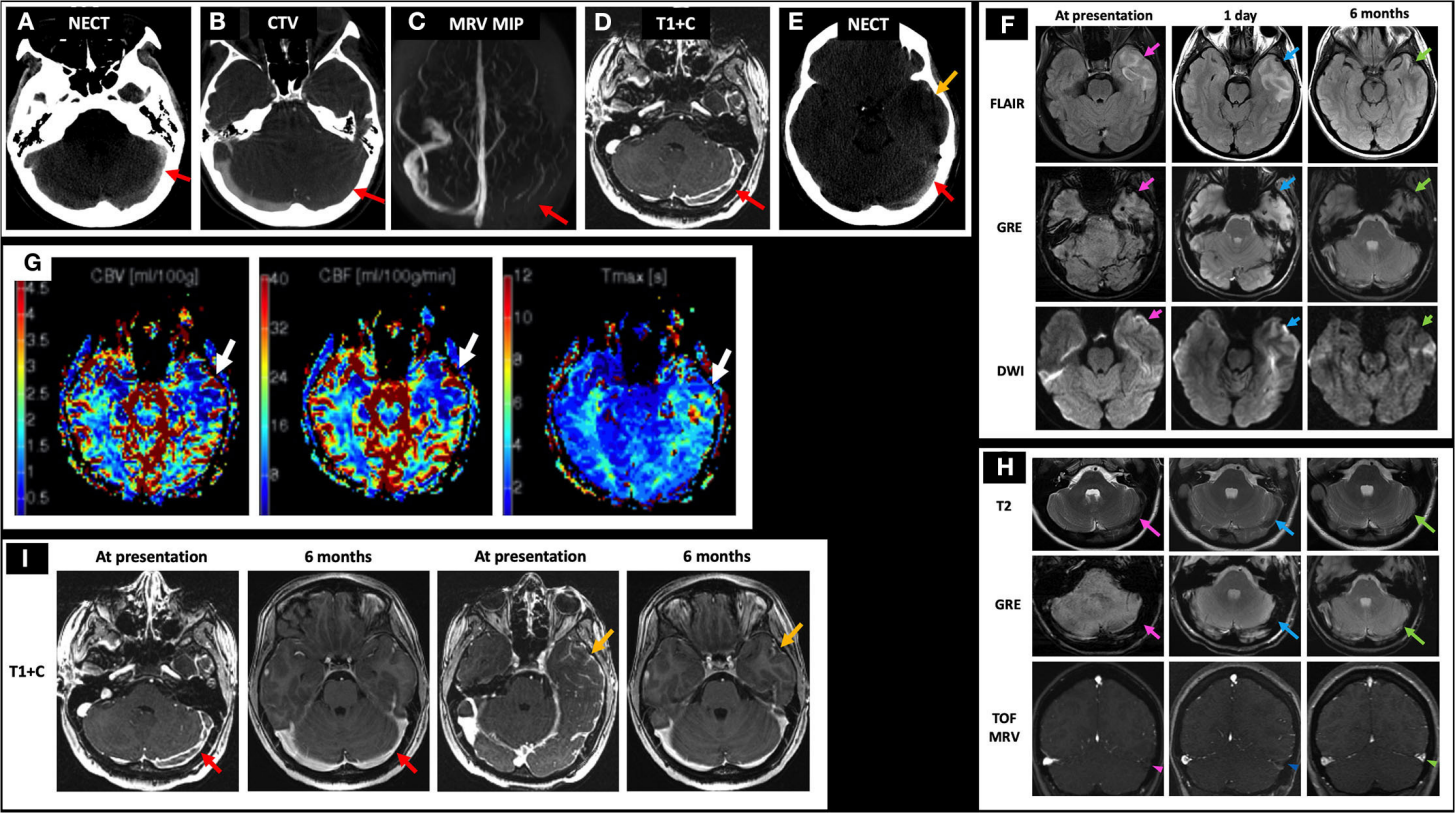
Case 3: A 19-year-old female with history of oral contraceptive use presented with 2 weeks of headache and witnessed seizures. Initial NECT showed hyperdense thrombus in left TS (A, red arrow), VL, and SS with associated filling defects on CTV and MRI/MRV (B–D, red arrows). One day after initiating anticoagulation, her NECT showed a left temporal hypodensity (E, yellow arrow) with MRI showing FLAIR hyperintensity, GRE hypointensity, and DWI hyperintensity (F, left column, pink arrows) but without corresponding ADC hypointensity (not shown). Bolus perfusion imaging showed decreased CBV and CBF and increased Tmax (G, white arrows). These findings represent vasogenic edema and petechial hemorrhage and thus ischemic but not infarcted tissue, consistent with CVT rather than CVI. Follow-up C-MRI/MRV 6 months later showed progression of the T2 hypointense thrombus to an isointense appearance (H, top row), improved susceptibility in the left TS (H, middle row), and resolved filling defects in the left TS on TOF MRV and C-MRV (H, bottom row; I, red arrows), as well as resolved left temporal gyriform enhancement (I, yellow arrows). On subsequent hypercoagulability work-up, she was found to have protein C deficiency. In (H), the pink arrows point to the left TS thrombosis at presentation on T2, GRE, and TOF MRV, respectively (left column). The blue arrows point to the left TS thrombosis at 1 day follow-up on T2, GRE, and TOF MRV, respectively (middle column). The green arrows point to the left TS thrombosis at 6 months follow-up on T2, GRE, and TOF MRV, respectively (right column). CBF, cerebral blood flow; CBV, cerebral blood volume; CTV, CT venography; C-MRV, contrast-enhanced MRI venography; MRP, MRI perfusion imaging; TOF MRV, time-of-flight MRI venography; NECT, non-contrast CT; OCP, oral contraceptives; Tmax, time-to-maximum; TS, transverse sinus; VL, vein of Labbe; SS, sigmoid sinus.
